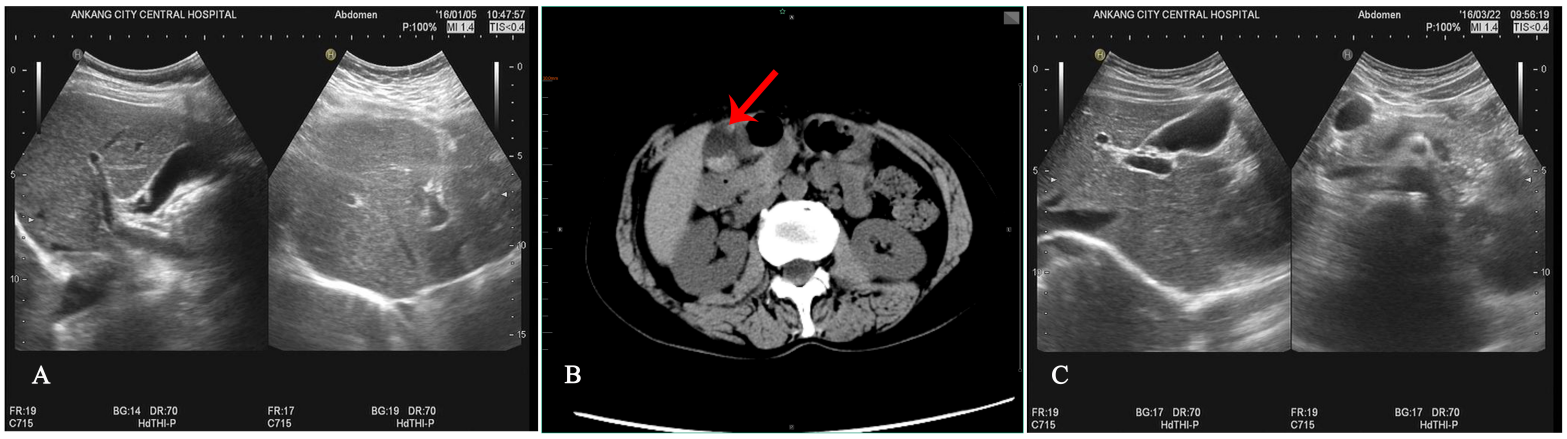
Results of auxiliary examinations. (A) Abdominal B-ultrasound before ceftriaxone sodium administration indicates a smooth gallbladder wall with no strong echogenicity in the lumen. (B) Abdominal CT after ceftriaxone sodium administration reveals a normal-sized gallbladder with a smooth wall and multiple nodular high-density shadows within. (C) Follow-up B-ultrasound 42 days after symptomatic treatment shows the disappearance of gallbladder stones.
